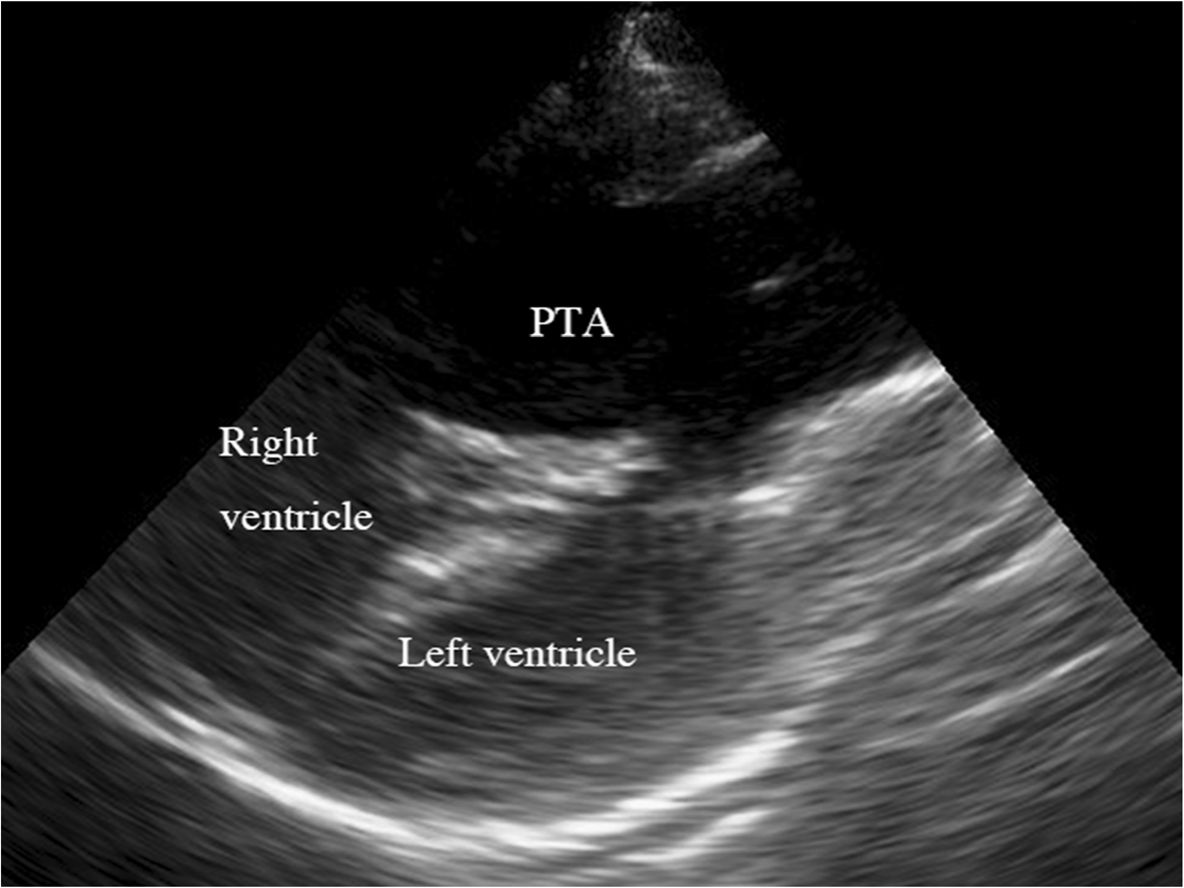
The image of echocardiogram. An echocardiogram revealed PTA overriding both ventricles
